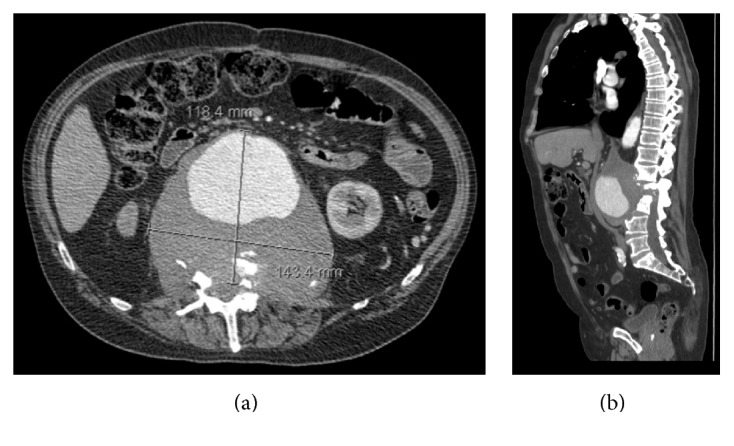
Single images from the CT angiogram scan of the perirenal saccular abdominal pseudoaneurysm with adjacent discitis. (a) Axial image of the pseudoaneurysm 11.8 × 14.3 cm in dimensions. (b) Sagittal image of the pseudoaneurysm with concomitant L1–L3 discitis.
